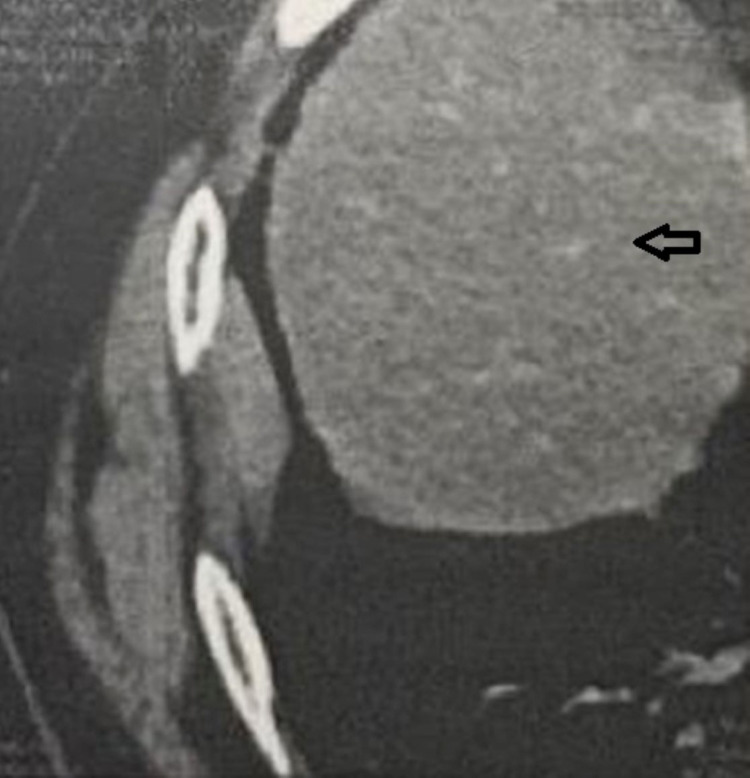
CT showing pleural metastasis of osteosarcoma
